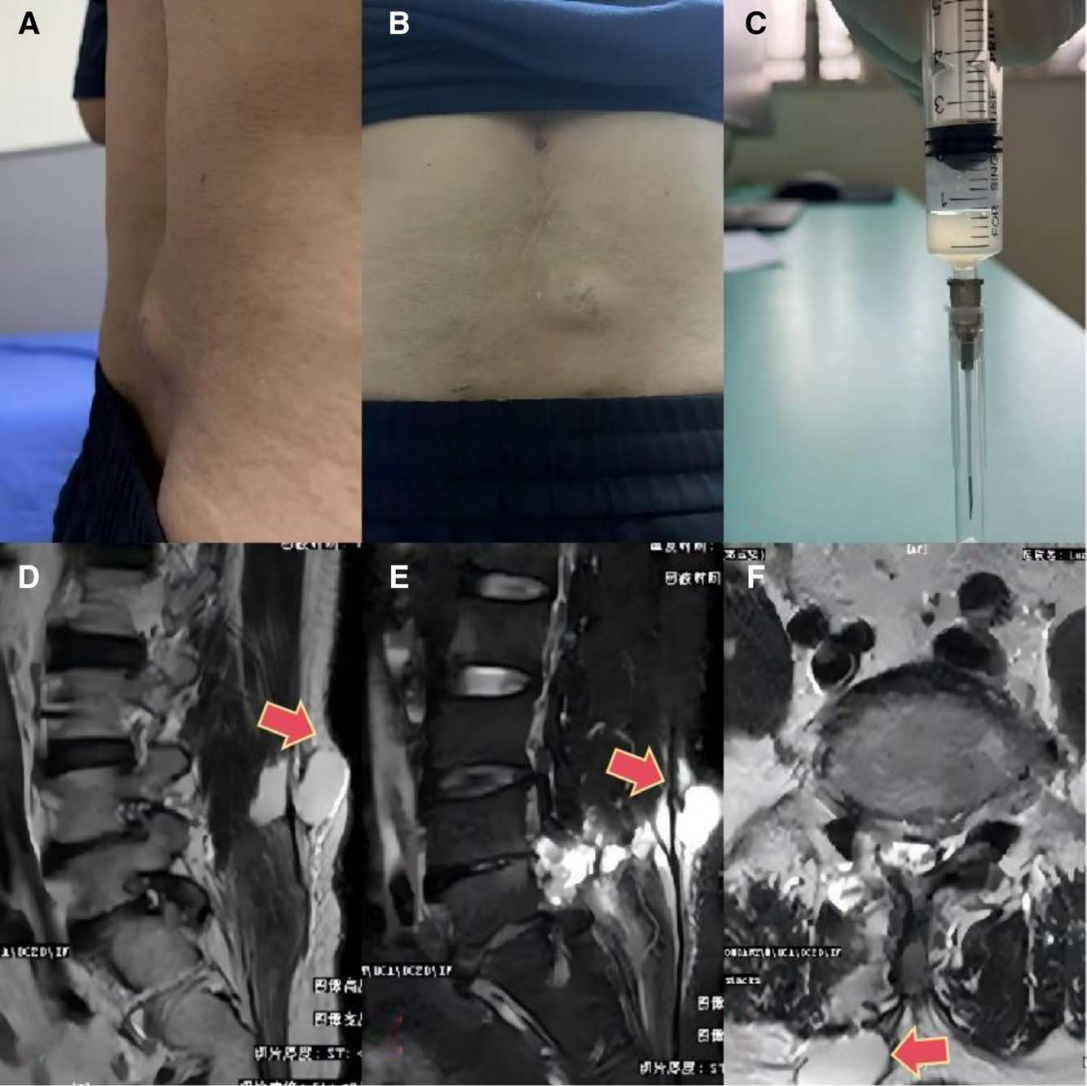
(A, B): Cystic masses were observed in the skin at the surgical area of the lumbar following UBE procedure. (C): Analysis of the puncture fluid from the lumbar mass revealed its properties.(D, E, F): MRI imaging demonstrateed subcutaneous accumulation of cerebrospinal fluid (CSF) at the L4-5 segments. The effusion was contiguous with the spinal canal. MRI = magnetic resonance imaging, UBE = unilateral transforaminal endoscopic spine surgery.
